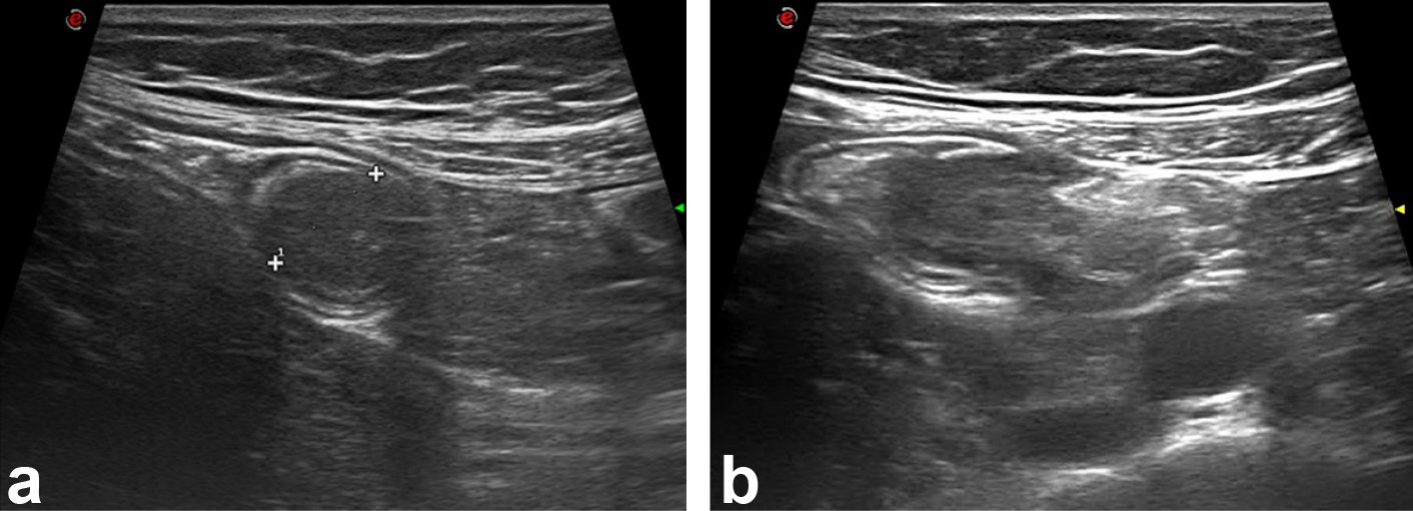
(a, b) Ultrasound revealed a homogenous hypoechoic and thick-walled tubular lesion with regular forms located at the pericecal area; no internal vascularity was noted.
